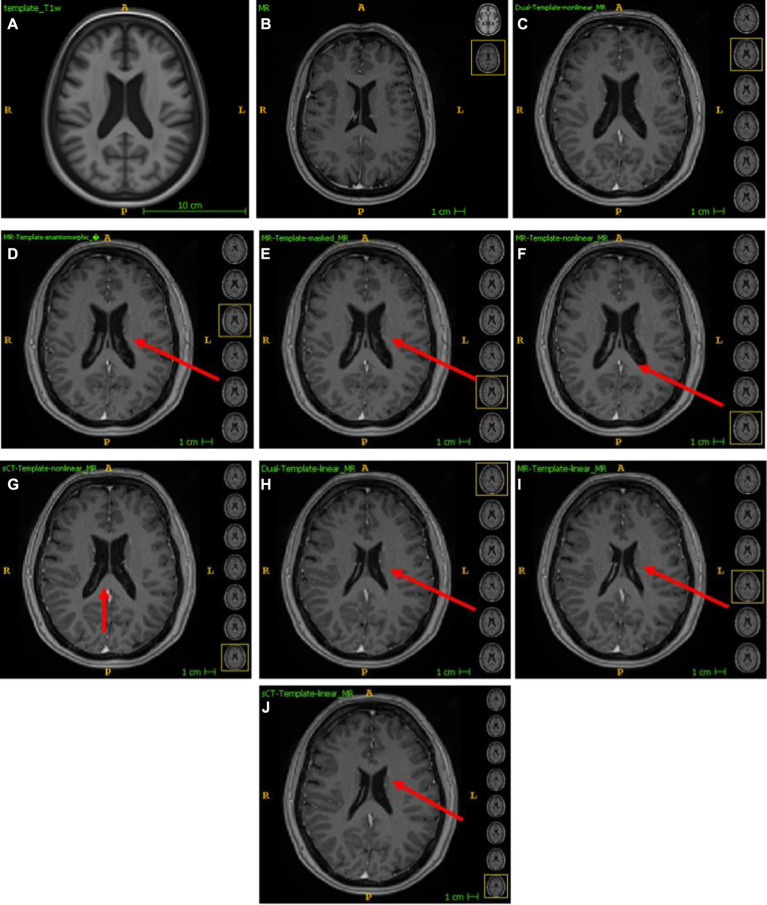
Comparison of axial slices of the MR images registered on to the template space (A), the native space (B) using the Dual-Template-non-linear (C), MR-Template-enantiomorphic (D), MR-Template-masked (E), MR-Template-non-linear (F), sCT-Template-non-linear (G), Dual-Template-linear (H), MR-Template-linear (I), and sCT-Template-linear schemes (J). The images are shown from highest to lowest score, with the Dual-Template-non-linear scoring a 5 and the sCT-Template-linear scoring a 2. The red arrows point to distortions in the shape and location of the ventricles.
